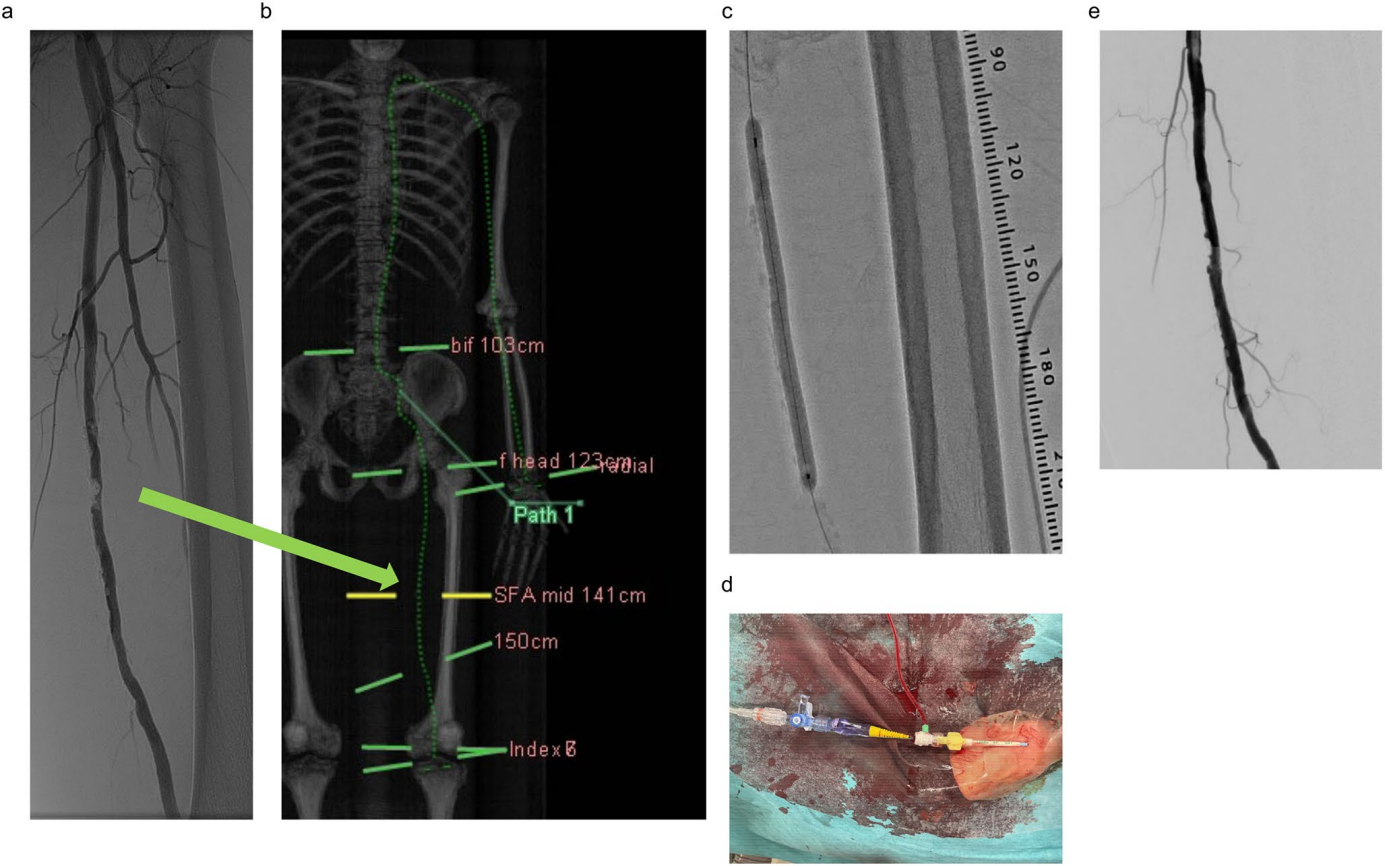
A case in which the puncture site was determined using preoperative CT. (a) Pre-operative angiographic image. (b) Pre-operative CT image. (c) Expanding DCB (Drug Coating Balloon) 5/100 cm. (d) Catheters outside the body. (e) Post-operative angiographic image
